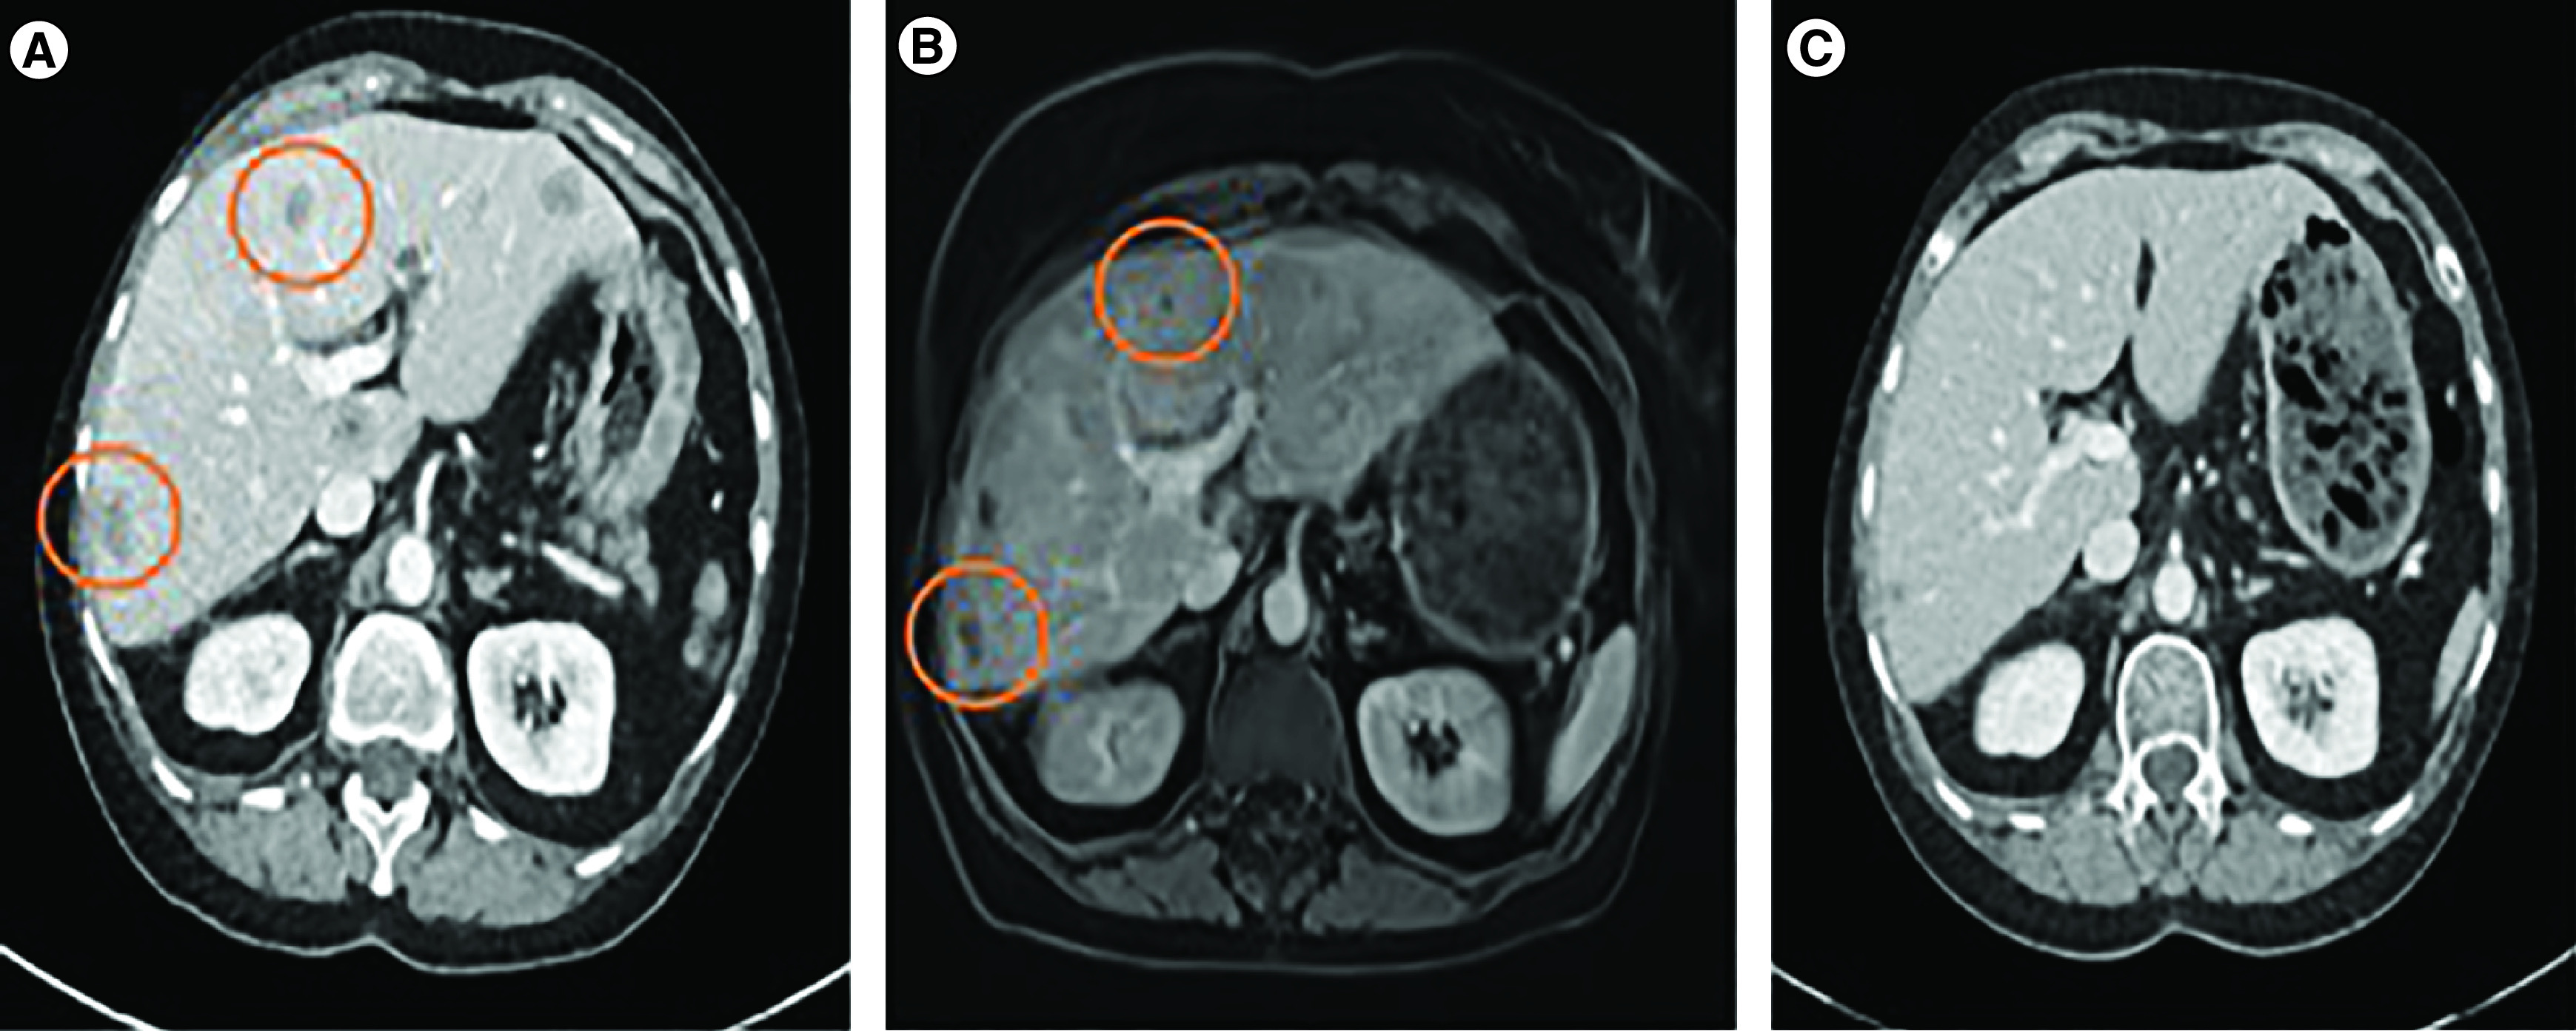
Evolution of liver metastatic lesions on baseline and after trans-arterial radioembolization. (A) Abdominal CT before TARE. (B) Abdominal MRI performed 2 months after TARE, showing decrease of all previously described lesions. ^18^FDG-PET did not show any metabolic lesion. (C) Abdominal CT performed 6 months after TARE, showing disappearance of previously described lesions. ^18^FDG-PET did not show any metabolic lesion. CT: Computed tomography; FDG: Fluoro-deoxy-glucose; TARE: Trans-arterial radioembolization.
